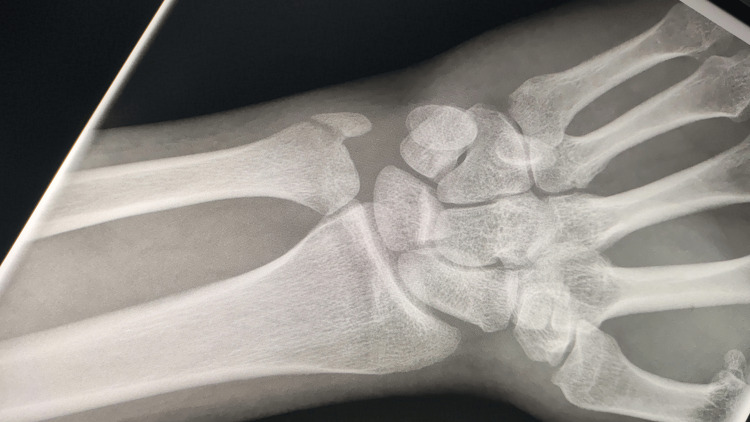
Follow‑up wrist X-ray Follow‑up wrist radiograph without cast showing maintained reduction and stable alignment of the distal radioulnar joint.
